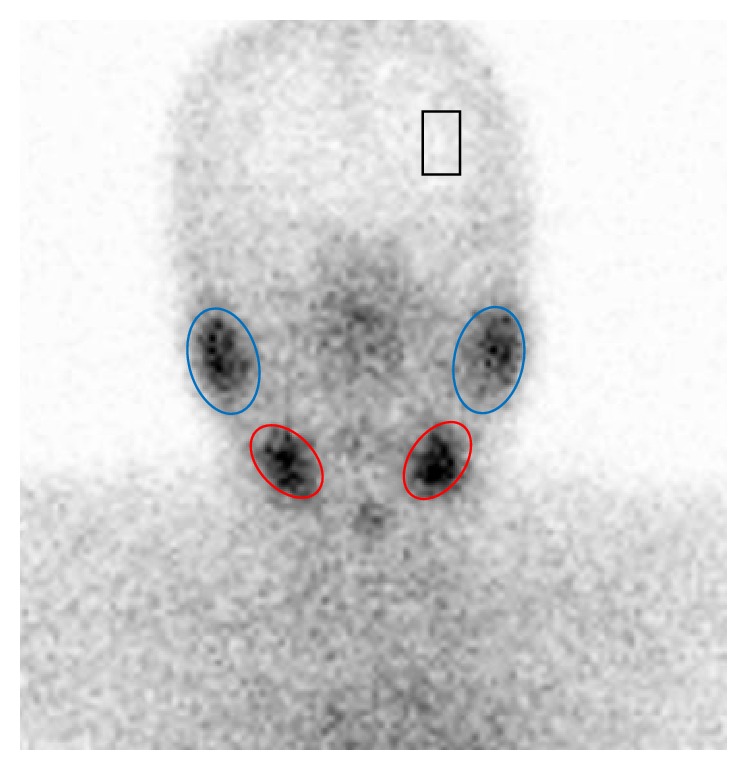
Regions of interest on the summation image as obtained by dynamic scintigraphy.
